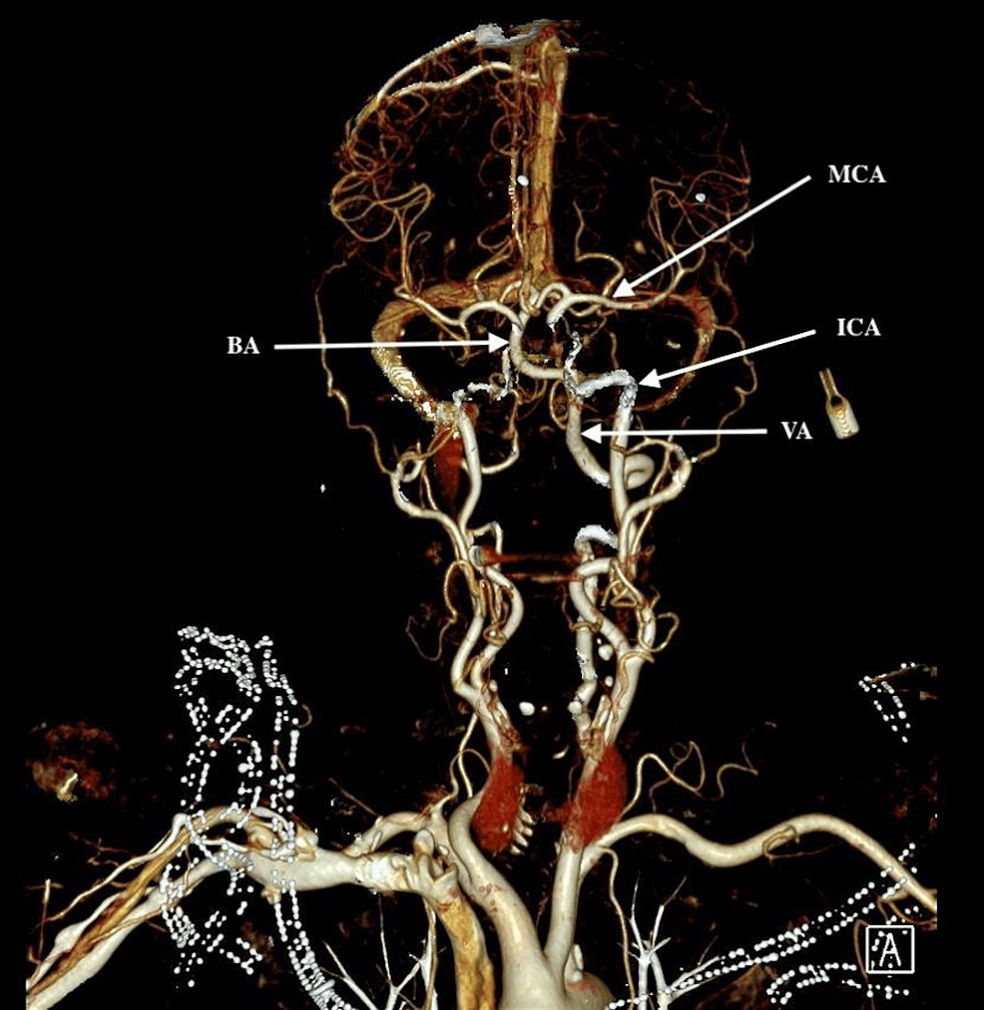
CT angiography, head, and neck major vessels are shown; no large vessel occlusion. BA: basilar artery, MCA: middle cerebral artery, ICA: internal carotid artery, VA: vertebral artery.
